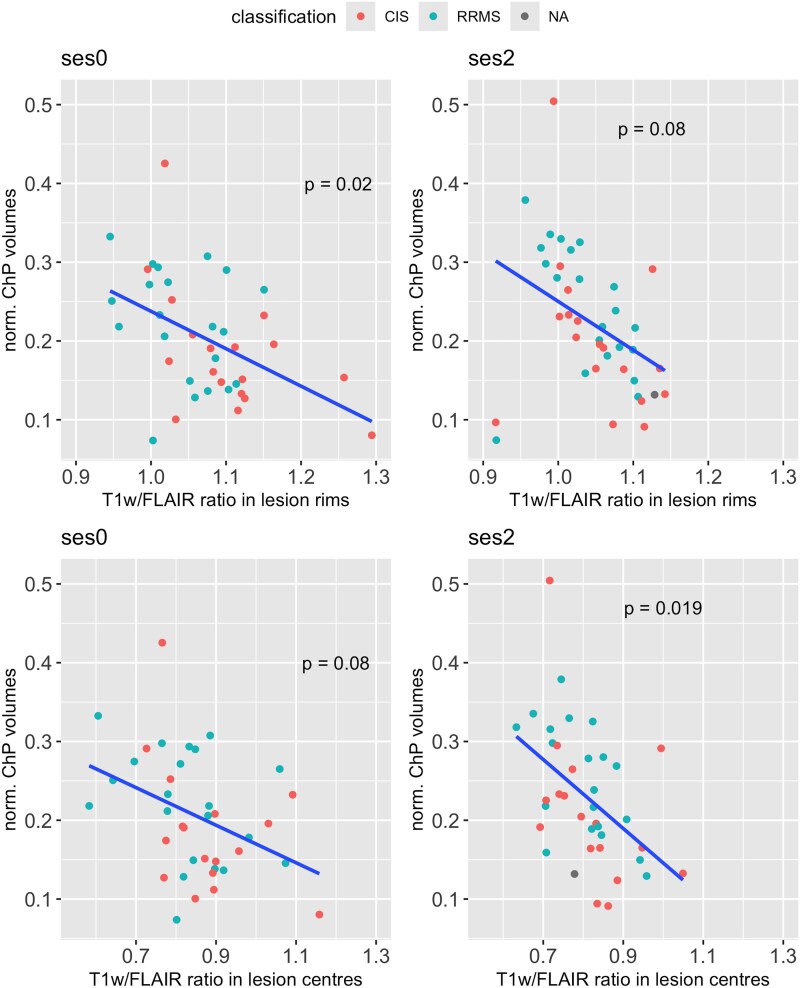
Choroid plexus volume versus lesion T1w/FLAIR ratios. Relationship between normalized ChP volumes and T1w/FLAIR ratios within lesion rims (upper row) or T1w/FLAIR ratios within lesion centres (lower row) at baseline (ses0) and at the last follow-up (ses2) (*N* = 39). Linear regression is represented by the bold line. *P*-values (for T1w/FLAIR ratio estimates) were derived from linear regression analyses including age (at baseline) and sex as covariates. CIS and RRMS patients (RRMS) are coloured in red and blue, respectively.
